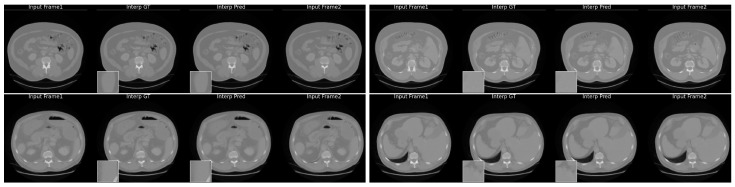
Qualitative visualization of liver CT inter-slice interpolation.
